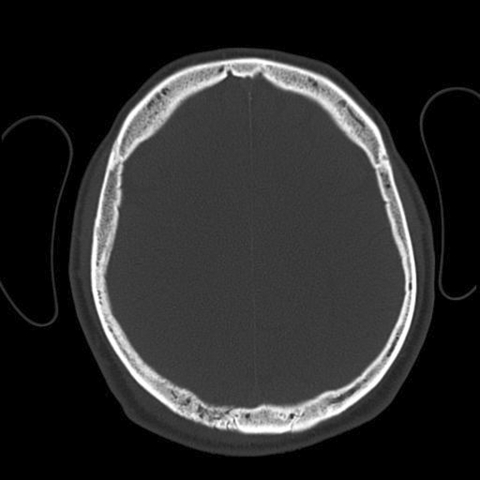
Axial nonenhanced computed tomography showing moth-eaten appearance of right parietal bone characteristic of osteomyelitis.
